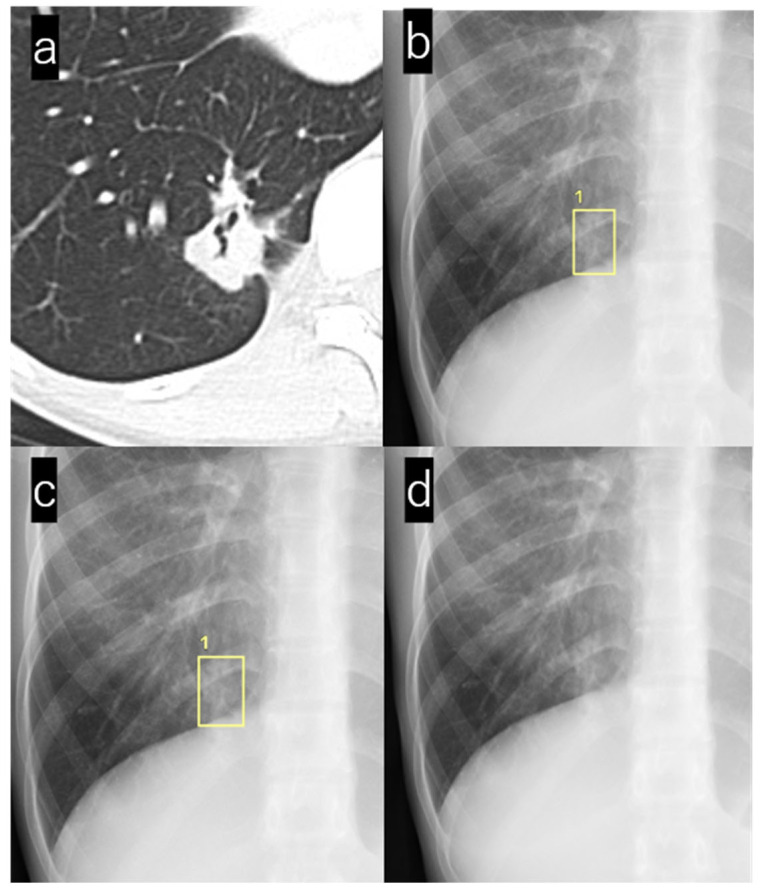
Computed tomography shows a 20 mm nodule in the lower-right lobe of the lung (a). The nodule is detected in the full-scale image (b,1) and the 10:1 irreversible compression image (c,1) when using AI detection of the chest radiograph. It is not detected in the 50:1 irreversible compression image (d).
